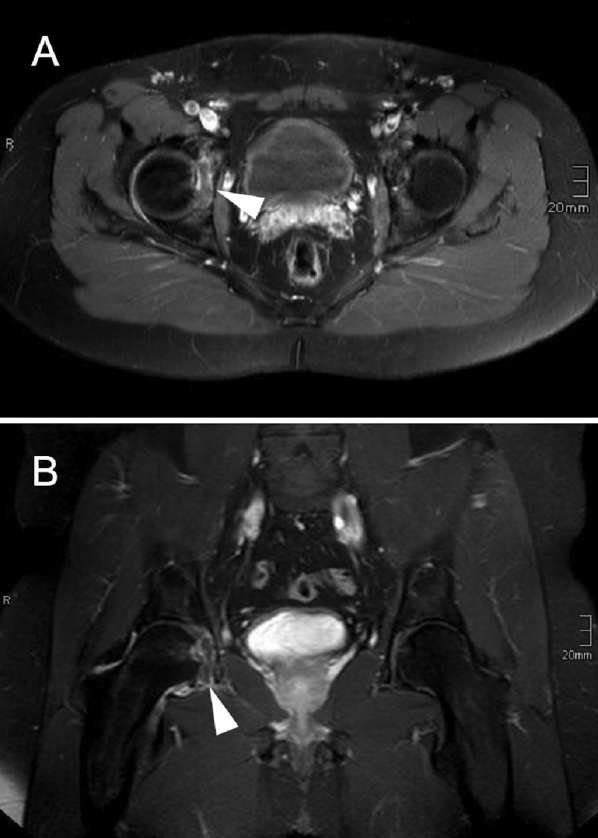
Gadolinium-enhanced MR images acquired with a fat-suppressed T1-weighted TSE sequence in transversal (A) and coronal orientation (B). Arrows show the minimally enhancing mass with a strong rim enhancement and a suggested relation to the caudal synovia. Notice the slight enhancement of the joint's capsule without the presence of effusion.
